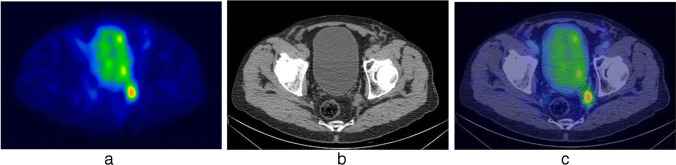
[^99^mTc]Tc-PSMA-I&S SPECT/CT scan identified a suspicious lymph node at the level of the left obturatory region, as shown in SPECT (a), CT (b) and SPECT/CT (c) axial view
